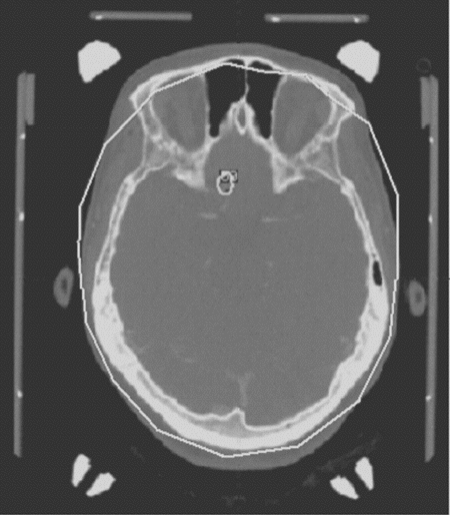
Skull model (solid white line) superimposed on a computed tomography image. Note the large discrepancy between the skull model and the actual patient contour in the anterior and posterior portions of the image.
